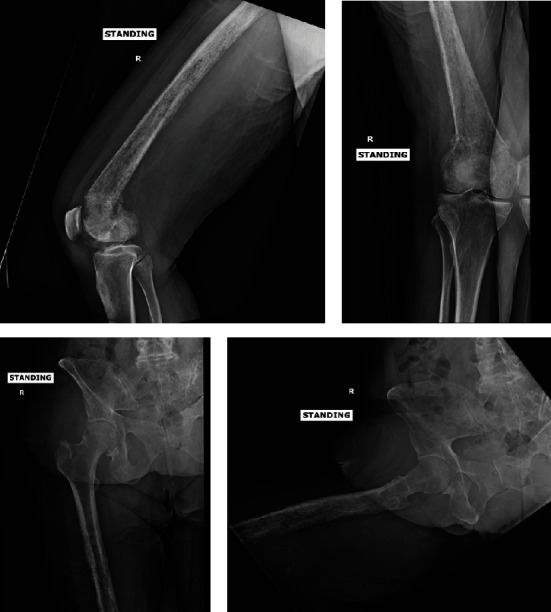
AP and lateral radiograph views of the femur revealing a healing, slightly dorsally angulated pathologic distal femur fracture with diffuse changes throughout the entire diaphysis.
